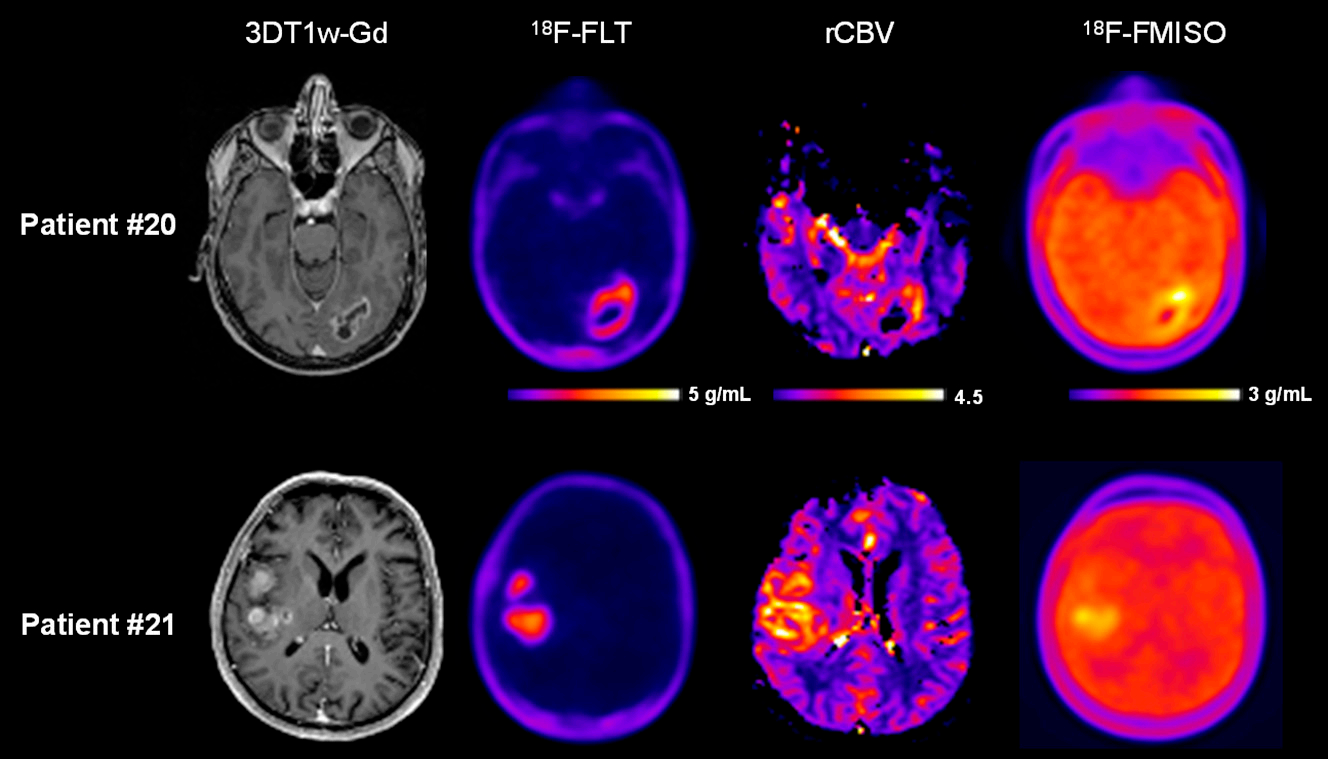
Multimodal imaging of 2 glioblastoma patients with 3D T1w-Gd, ^18^F-FLT PET, rCBV MRI, and ^18^F-FMISO PET.
